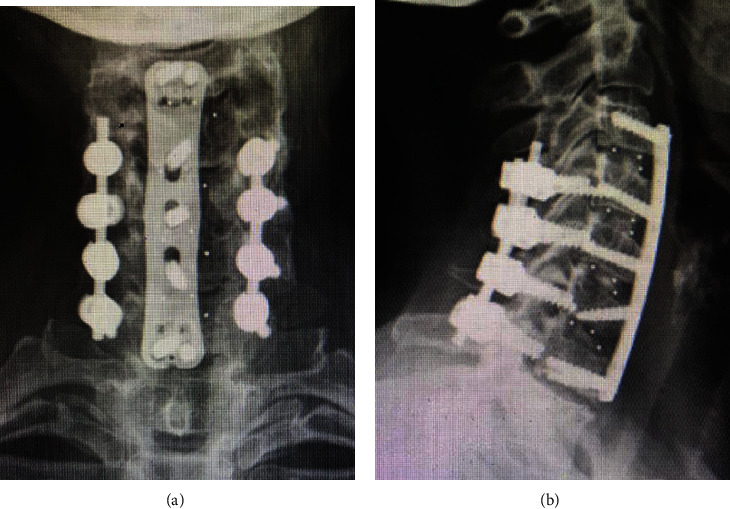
X-rays showing the alignment of the cervical spine using plates and screws. (a) PA view. (b) Lateral view.
